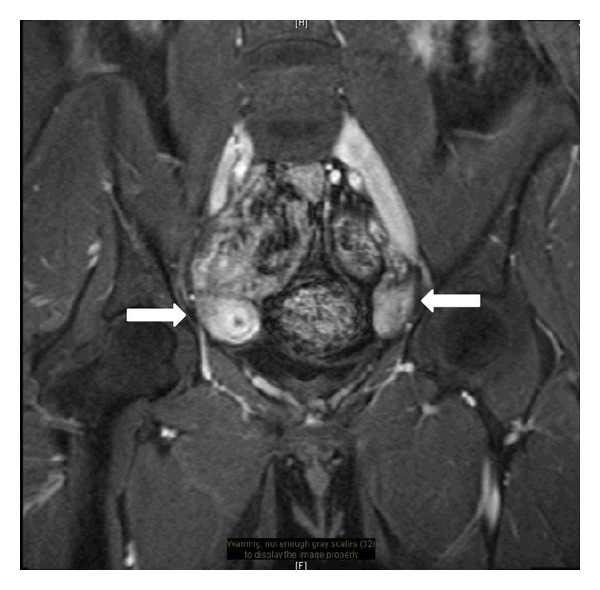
Coronal magnetic resonance imaging (MRI) scan showing two masses seen at bilateral pelvic sidewalls demonstrating enhancement patterns suggestive of possible uterine tissue (arrows). The right and left masses measured 3.2 × 1.9 cm and 2.9 × 1.6 cm in the maximum dimensions, respectively.
